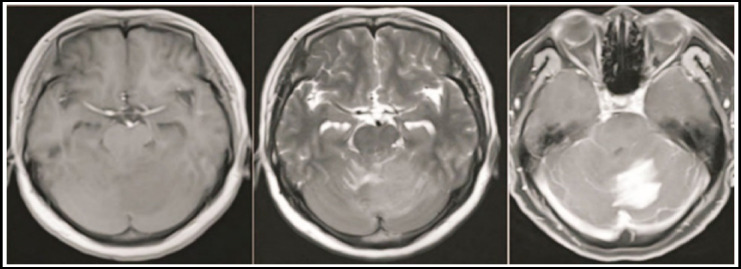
MRI image of diffuse large B-cell lymphoma.
